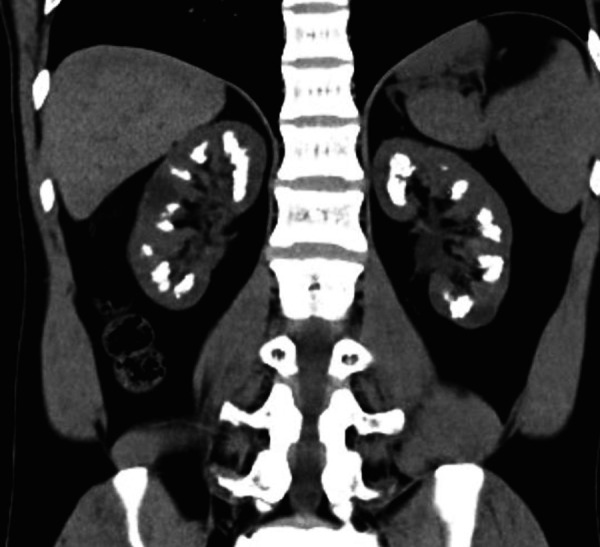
Abdomen CT (coronal view) showing medullary nephrocalcinosis.
